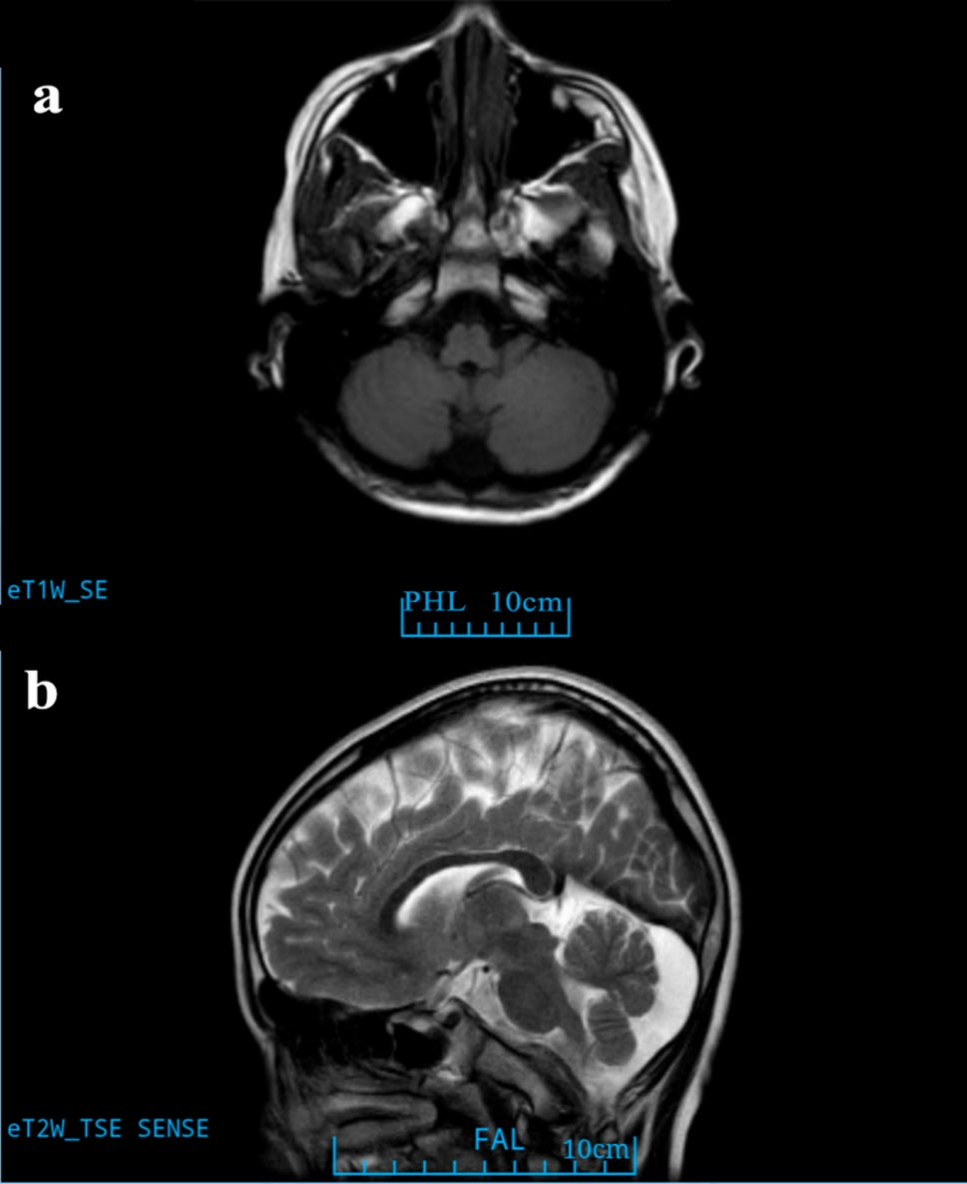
1.5T MRI (a) axial T1 and (b) sagittal T2 image showing mega cisterna magna at the age of 10
